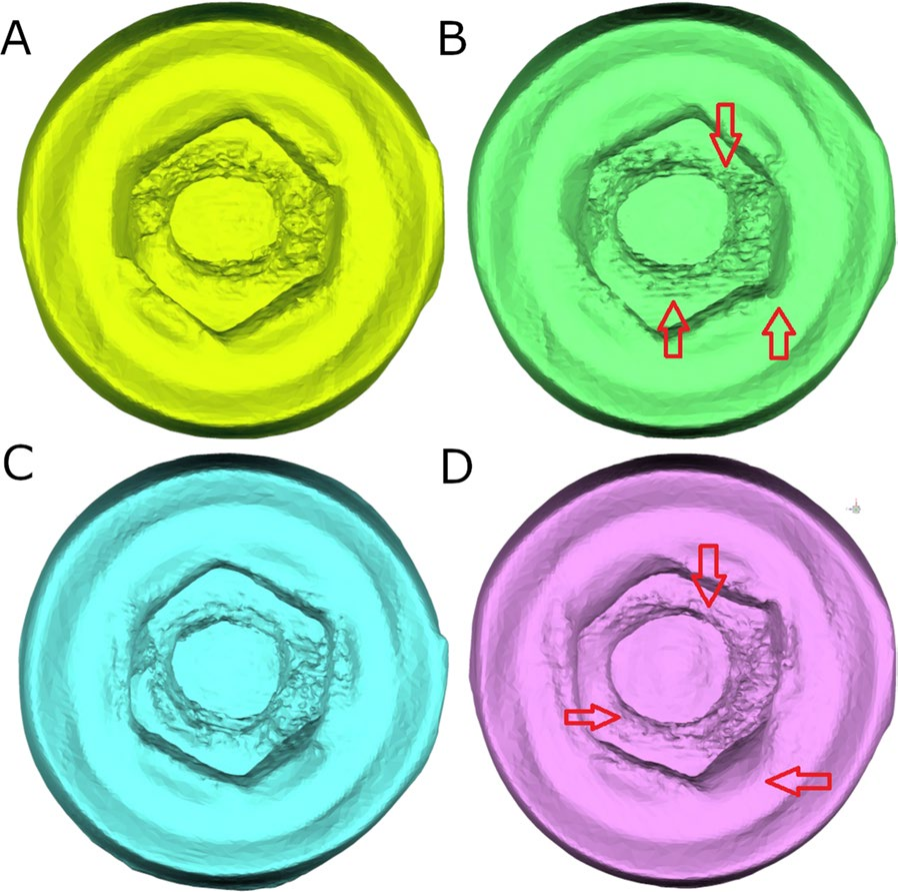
A Dental implant connection view of the preoperative and B postoperative STL digital files after removing the fractured abutment screws with drilling technique without irrigation. C Dental implant connection view of the preoperative and D postoperative STL digital files after removing the fractured abutment screws with ultrasonic technique without irrigation. The red arrows indicate the areas of wear
